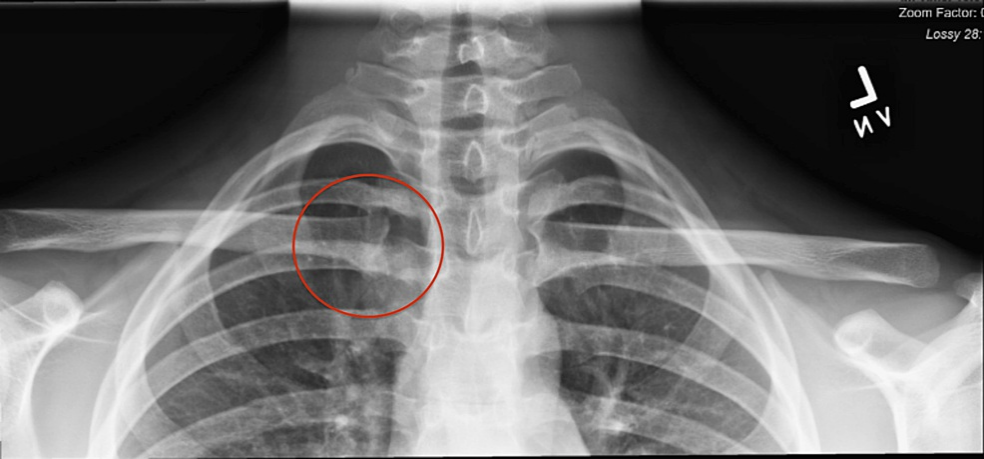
X-Ray: Anterior-Posterior View Red circle: right sternoclavicular joint
